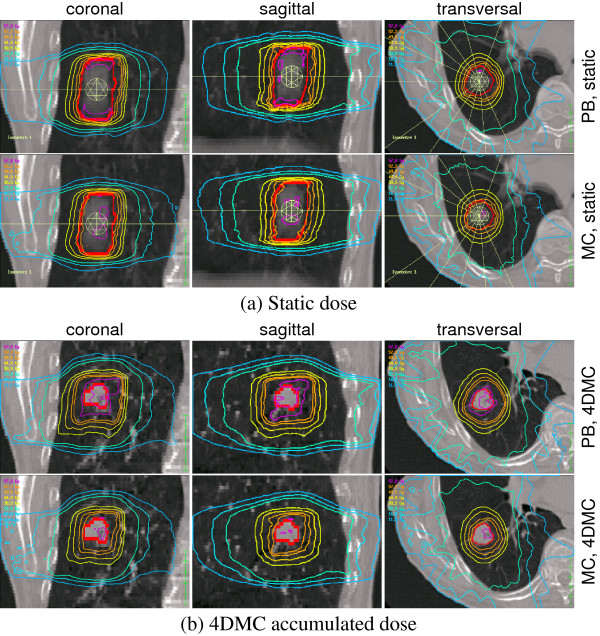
Dose distribution isodoses. Example of the resulting dose distributions from optimization with fsPB and MC with 6 MV beam energy and using the average CT patient model (a). The same plans recalculated with 4DMC (b).
